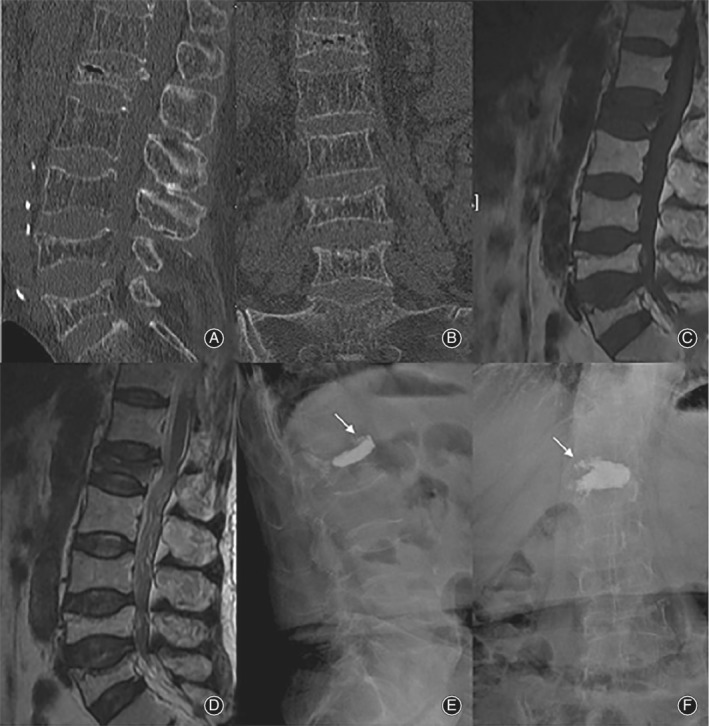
A 65‐year‐old‐female patient who underwent PKP for L_1_ Kummell disease. (A and B) Preoperative CT scan showing L_1_ fracture with IVC, and MRI showing IVC on the T1(C) and T2‐weighted (D) images. Postoperative X‐rays (E and F) revealing radiographic results, which shows cement leakage at T_12_ L_1_ disk space (arrow).
